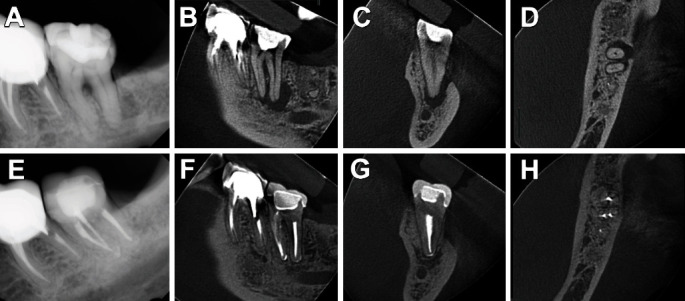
Comparative radiograph and CBCT images performed before (A–D) and after 2 years of treatment of tooth #18 revealed apical repair and the absence of crestal bone loss (E–H).
